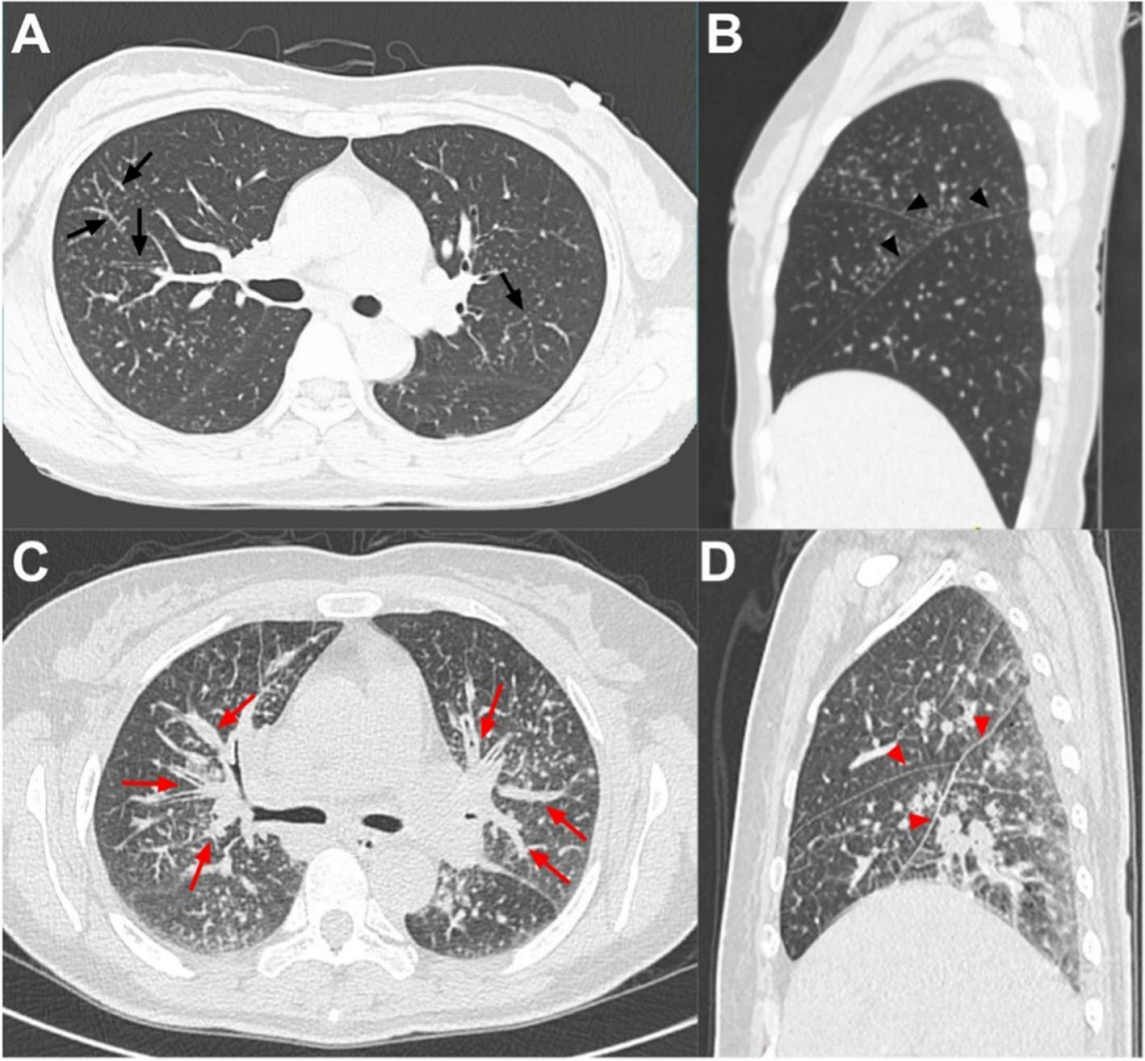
A, B, Axial CT and sagittal CT image in a 46-year-old endometrial cancer woman with pulmonary sarcoidosis showed bronchovascular bundle (black arrows) and pleural nodular thickening (black triangle). C, D, Axial CT and sagittal CT image in a 54-year-old ovarian cancer woman with pulmonary lymphangitic carcinomatosis showed bronchovascular bundle (red arrows) and pleural diffuse thickening (red triangle)
